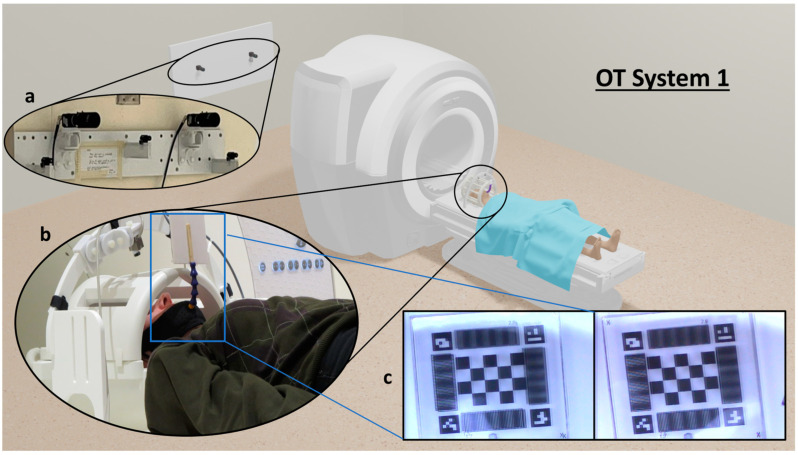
OT System 1. (a) Two cameras are mounted on the rear wall in the magnet room facing the magnet bore. (b) A participant lies in the head coil, who is fitted with a wearable device with a fiducial marker attached. (c) The marker is visible in each camera as displayed in the bottom right. OT = Optical Tracking. This figure includes 3D assets adapted from “Sci-Fi MRI” by Mihail Shegolev (https://skfb.ly/oESMO accessed on 6 May 2024) and “Human” by Aaron Kalvin (https://skfb.ly/6Z8LI accessed on 6 May 2024) licensed under CC BY 4.0.
